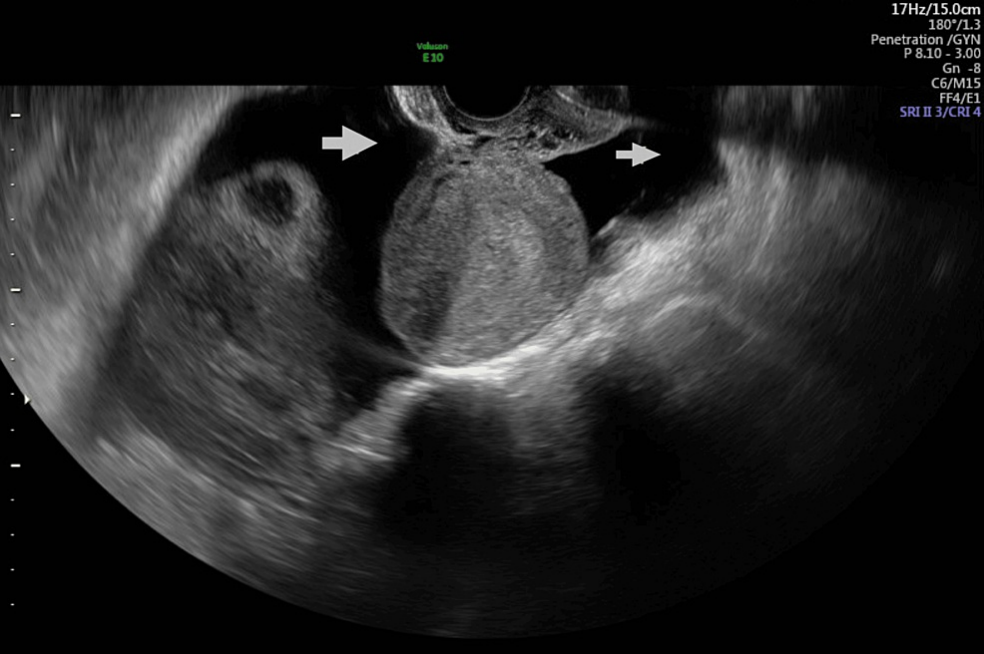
Transvaginal ultrasound during ovarian hyperstimulation Arrows show free fluid in the pouch of Douglas
